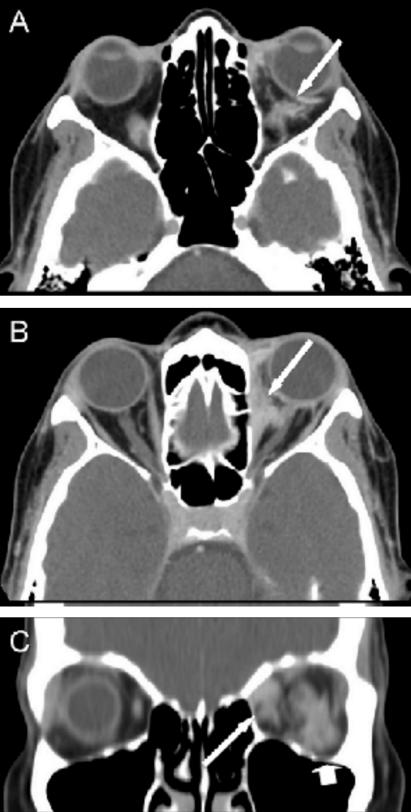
Retrobulbar metastasis, axial, and coronal computed tomography images with contrast. (A) Axial view of the inferior orbits demonstrates enhancing abnormal soft tissue posterior and lateral to the left globe (arrow). (B) Axial view of the upper orbits demonstrates enhancing abnormal soft tissue in the medial left orbit inseparable from the medial rectus muscle (arrow). (C) Coronal view demonstrates abnormal soft tissue in the medial left orbit inseparable from the medial rectus (arrow) and in the lateral inferior left orbit inseparable from the inferior rectus and in contact with the optic nerve (arrowhead).
